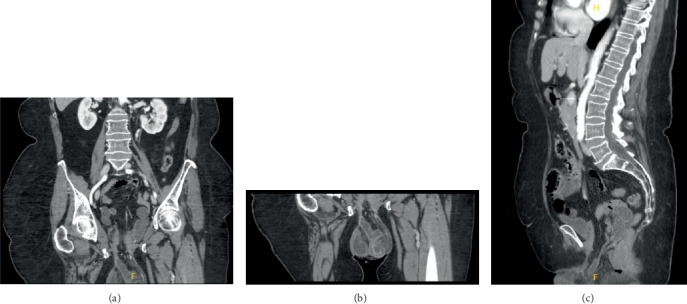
(a) Coronal CT imaging of the abdomen and pelvis. (b) Coronal CT imaging of the pelvis. (c) Sagittal CT imaging of the abdomen and pelvis.
